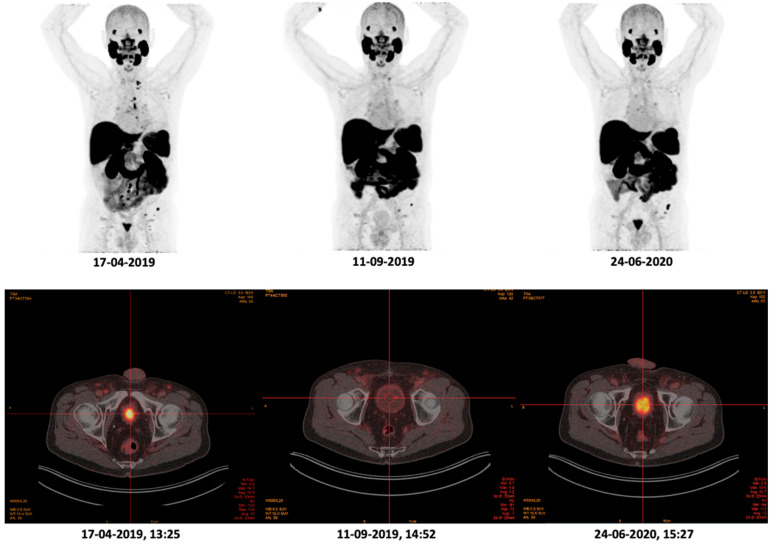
Maximum intensity projections and fused images of the urinary bladder uptake on [18F]PSMA-1007 PET/CT. This patient had an SUVmax (of the bladder) and bladder volume of 15.7 and 24 mL, respectively, in the first scan (17 April 2019), an SUVmax of 2.0 and bladder volume of 109 mL in the second scan (11 September 2019) and an SUVmax of 13.6 and bladder volume of 54 mL in the third scan (24 June 2020). Between these PET/CT scans, there were no changes in the patients’ medication use, blood laboratory results or introduction of new risk factors. PSMA: prostate-specific membrane antigen; PET: positron emission tomography; CT: computed tomography; MIP: maximum intensity projection; SUV: standardized uptake value; PSA: prostate-specific antigen.
